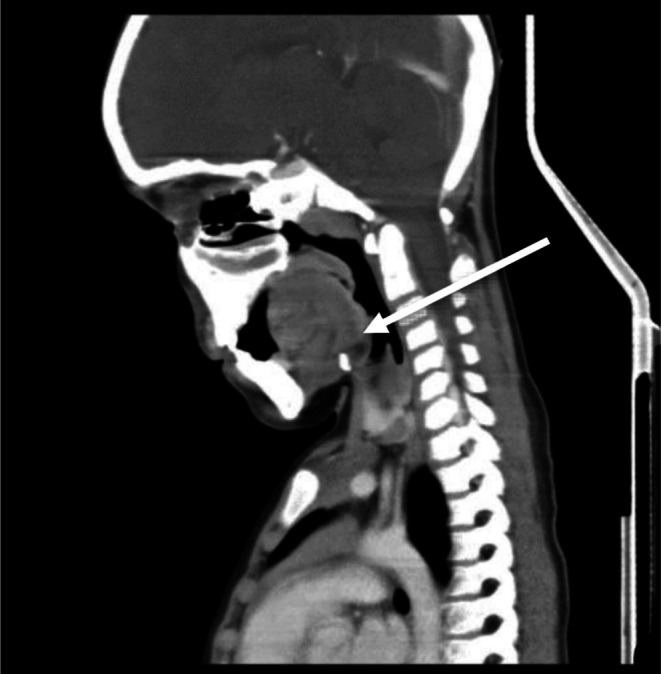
Axial CT image highlighting enlarged left cervical lymph node.
